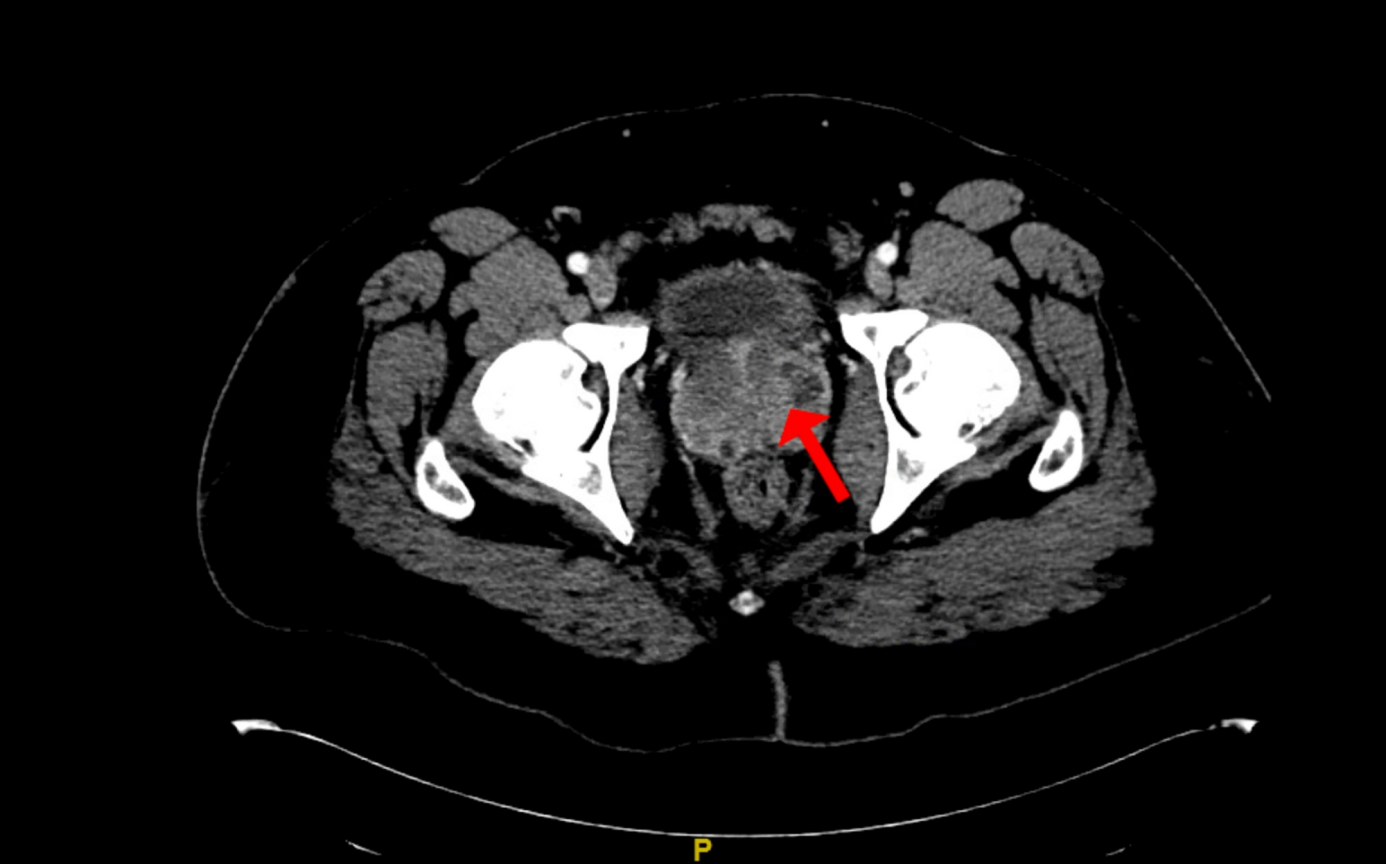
CT of the abdomen/pelvis with contrast demonstrating a moderately enlarged, heterogeneous appearing prostate gland with multiple hypoattenuating foci within it and surrounding inflammatory change concerning for multifocal prostatic abscesses.
